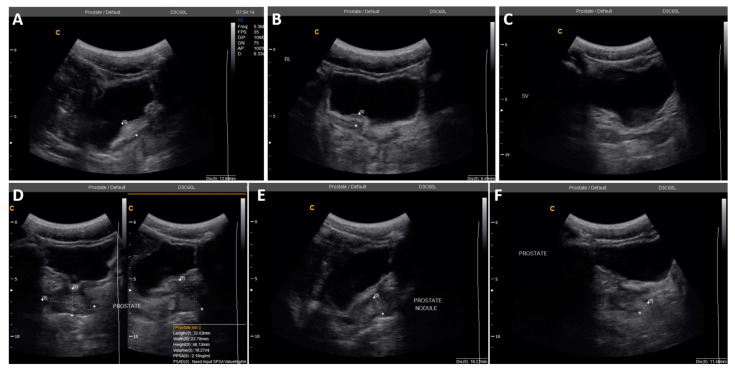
Ultrasonographic images of an MGS positive study participant with abnormalities in the bladder and prostate at Baseline. (A,B). Irregular urinary bladder wall and severe focal thickness, measuring up to 13.6 mm. (C). Normal symmetrical seminal vesicles. (D). Prostate with abnormal irregular outline, but normal volume of 18.3 mL. (E,F). Hyperechoic nodule in the prostate, measuring 11.4 mm by 16.2 mm.
